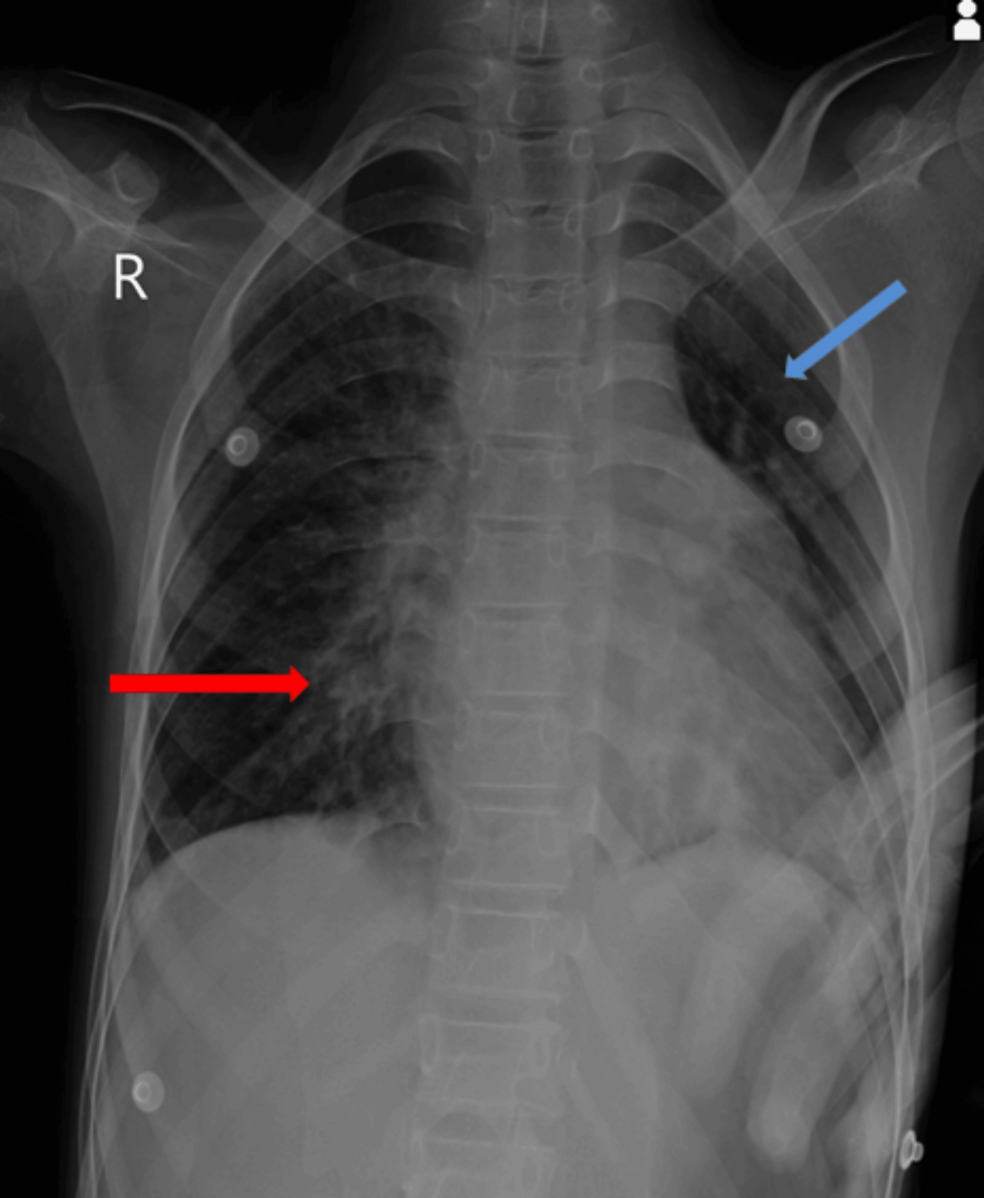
Chest X-ray showing right lung consolidation (indicated by red arrow) and left lung collapse (indicated by blue arrow)
